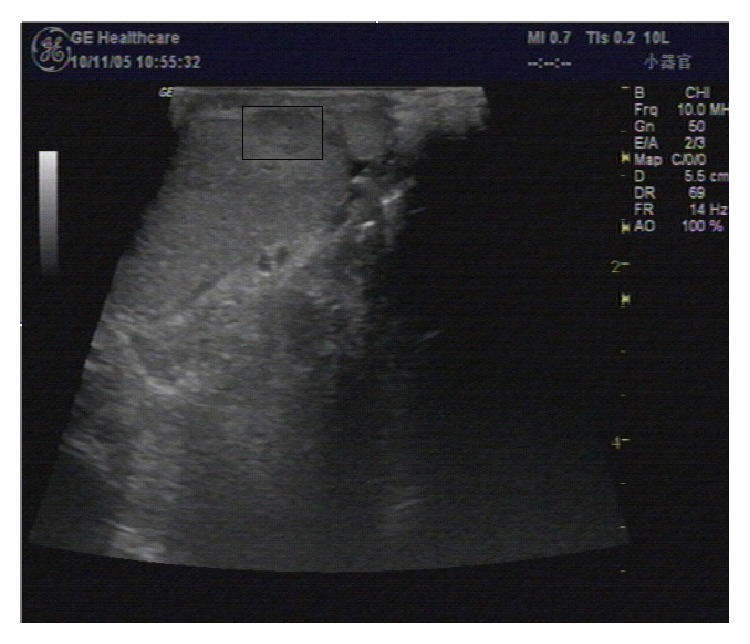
Scrotal ultrasonography of AT: scrotal ultrasound scan revealing a 10 × 8 mm, hyperechoic solid mass in the upper pole of the left testicle.
